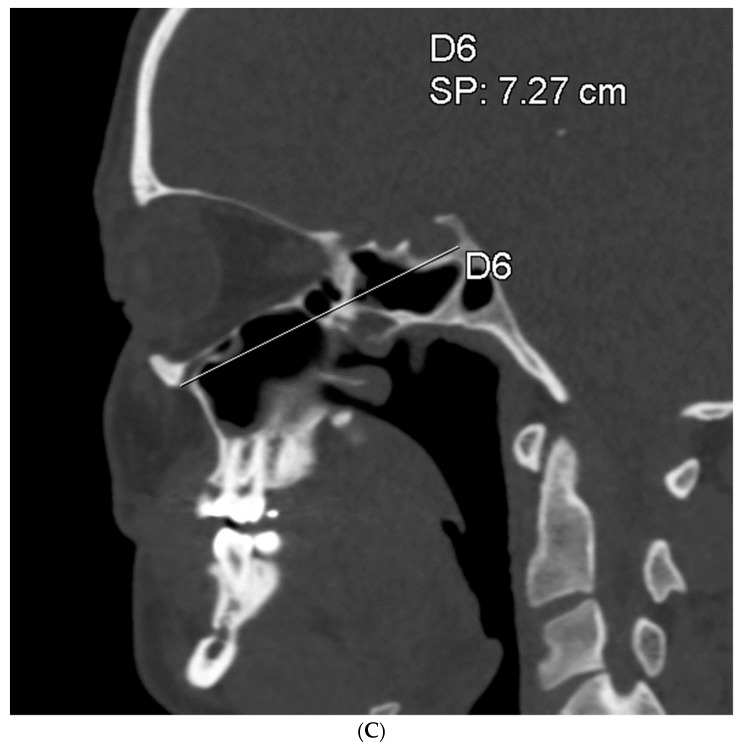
(A) The width and height of the bony orbit from the most anterior CBCT or CT slice showing the whole orbital rim. (B) The depth from the frontozygomatic suture to the optic canal opening. (C) The position of bony orbit was assessed as the distance of the cranio-lateral border of the infraorbital canal opening to the inner posterocaudal wall of sella turcica, measured from an oblique sagittal plane.
